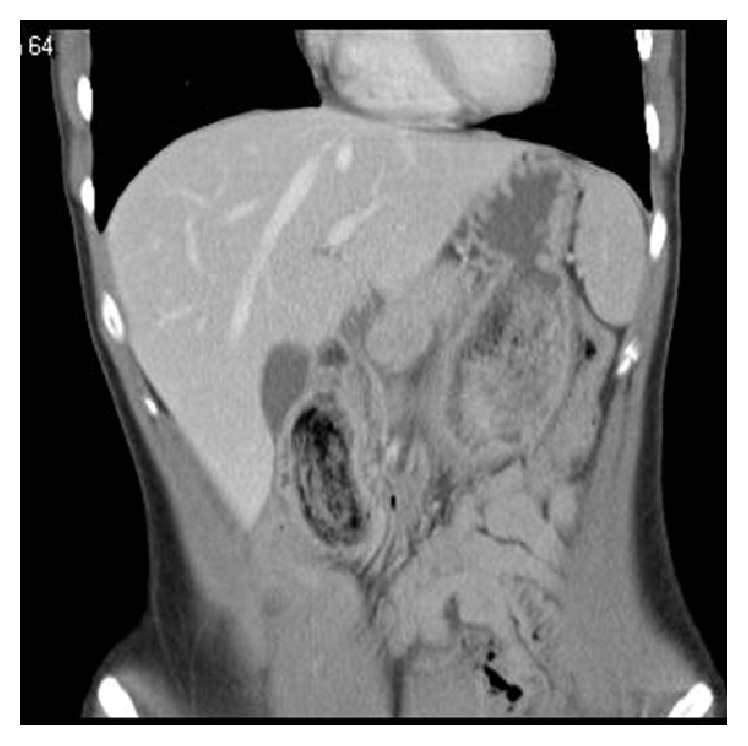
CT image showing bezoar extension from stomach into duodenum.
